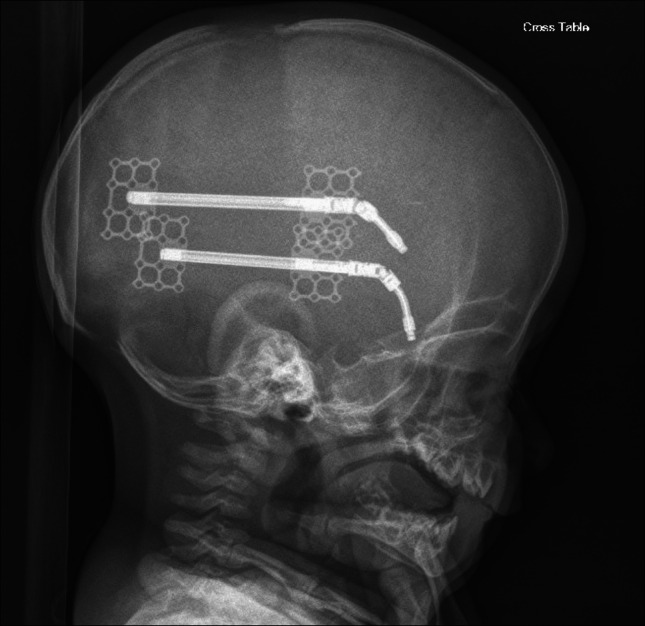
Lateral radiograph taken at consolidation showing robust posterior cranial vault expansion
